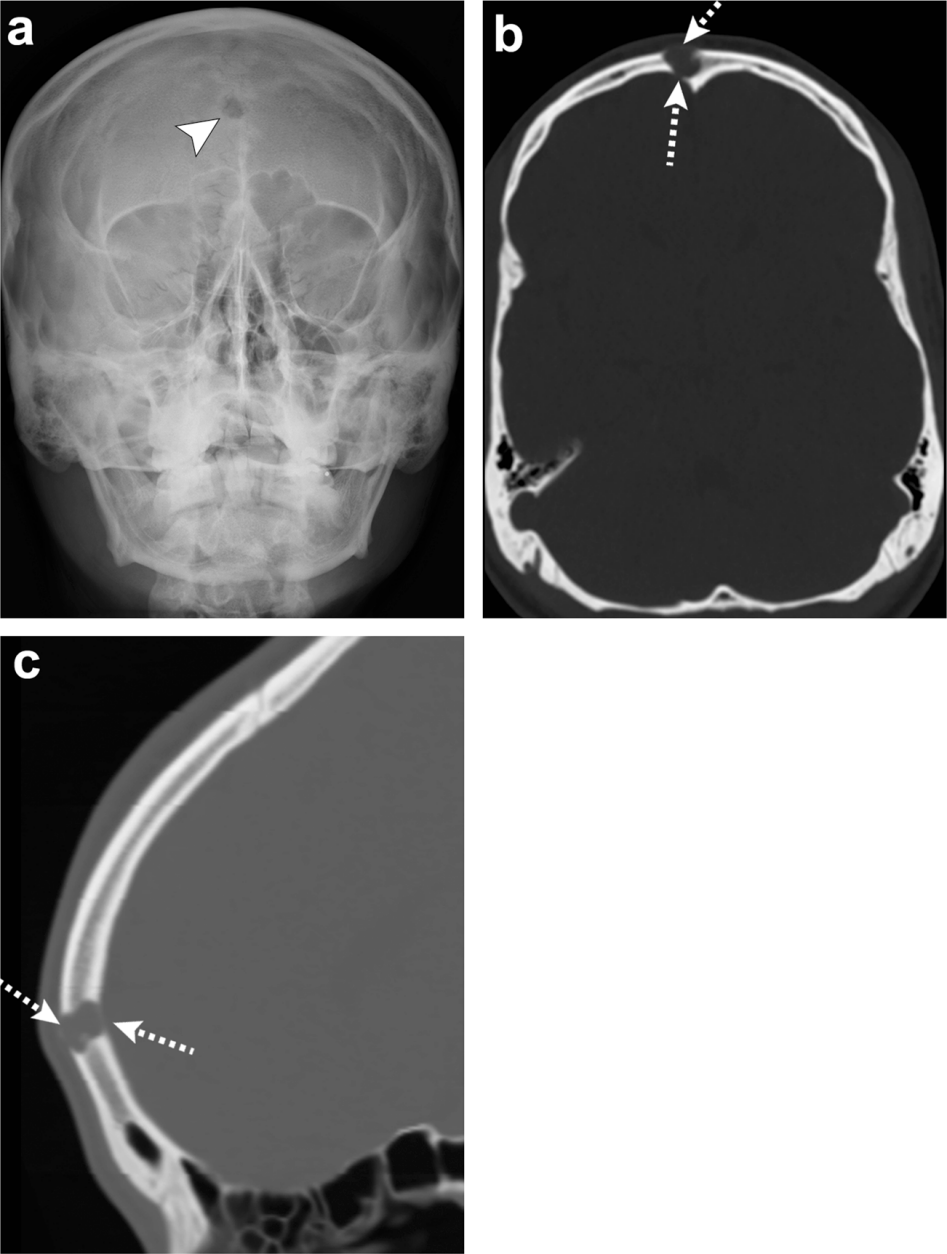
Ossifying fibroma. Water view skull radiograph (a), axial (b) and sagittal (c) head CT images show a lytic lesion in the frontal bone (*arrowhead*) with barely perceptible anterior and posterior cortical margins (*dashed arrows*)
